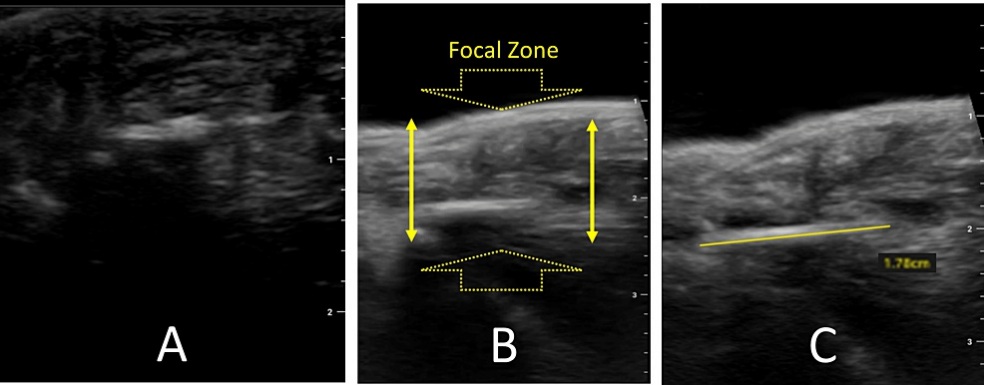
The focal zone advantage through a water bath A) Ultrasound probe on skin with gel and difficulty detecting the hyperechoic horizontal FB at 8 mm depth. B) Image of the same FB, slight oblique lay, in a water bath marking the focal zone. C) Total depth of the image reduced and probe adjusted, placing FB in true long axis, improving measurement accuracy. Note: posterior shadowing and some anechoic fluid create an early "halo sign" seen in images B and C yet indiscernible in image A.
